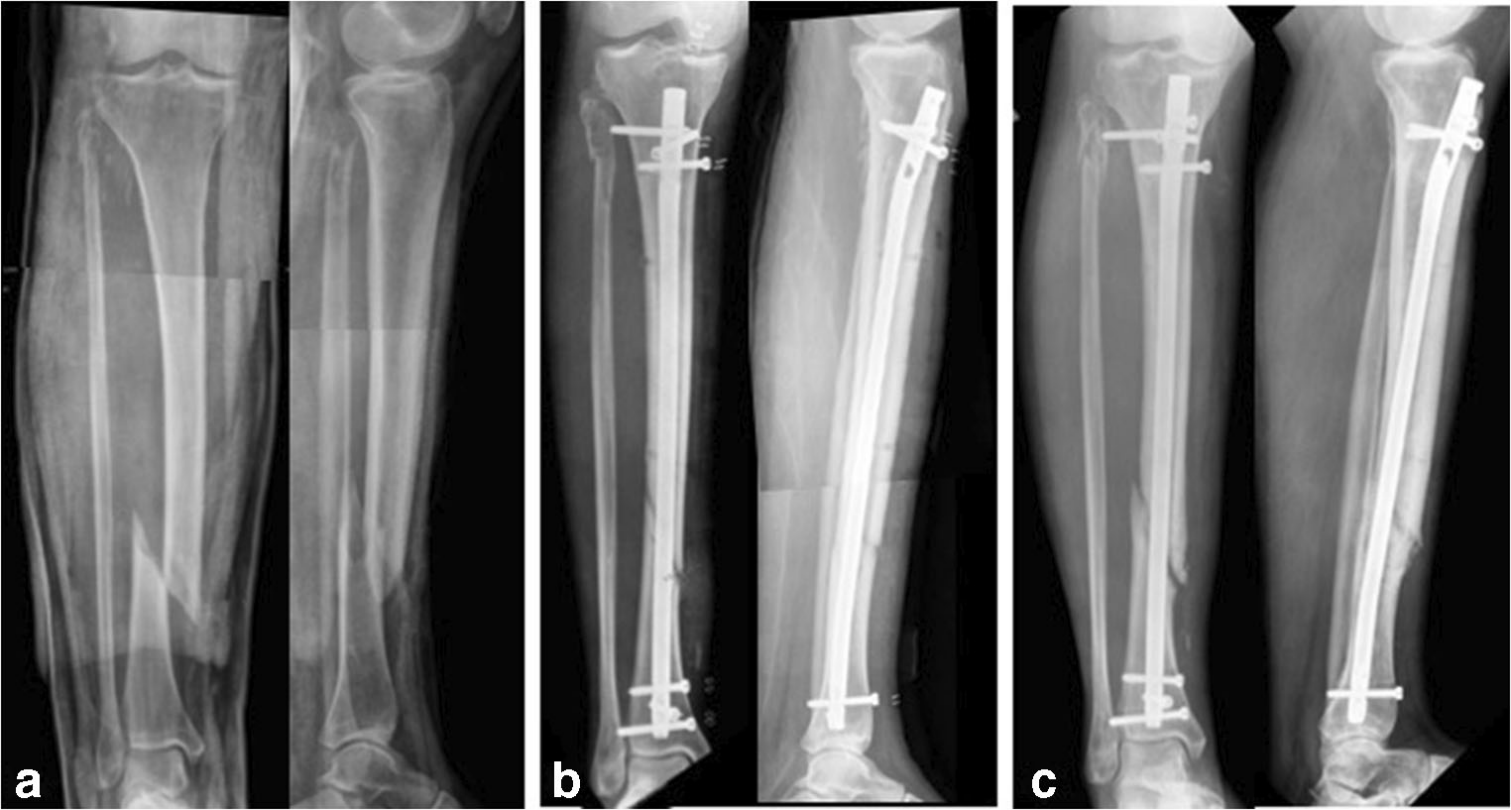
Patient 1: a Preoperative x-rays of distal tibial fracture. b Post-operative x-rays with intramedullary nail fixation. c 8-month follow-up films showing distal tibial non-union. The score calculated was 7 points (see Table 7)
